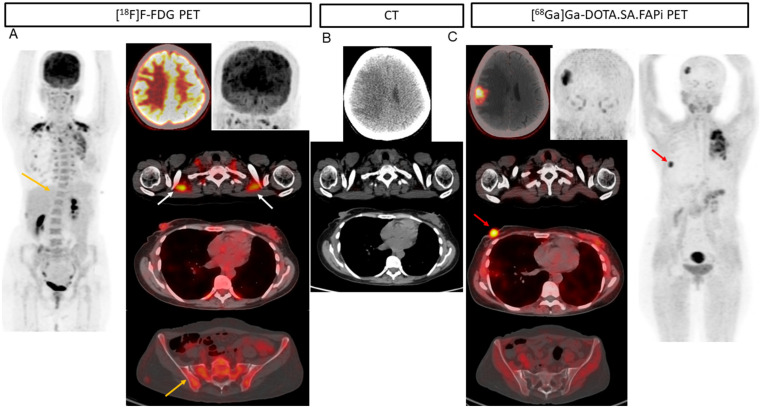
(A) 37-year-old patient with infiltrating ductal carcinoma underwent chemotherapy, Herceptin treatment, gamma knife for brain metastases, lapatinib, and capecitabine oral chemotherapy. A heterogeneous lesion measuring 1.7 × 1.4 cm was seen in the right parietal lobe associated with grade II perifocal vasogenic edema (B). (A) Due to the physiological uptake of FDG in the brain, lesions are not well demarcated (A). On [^68^Ga]Ga-DOTA.SA.FAPi PET scan, brain metastasis is appreciated in the MIP image itself (C). Brown fat uptake is seen on [^18^F]F-FDG ((A), white arrows), but no such findings were noted on [^68^Ga]Ga-DOTA.SA.FAPi PET scan (C). High-intensity uptake on [^68^Ga]Ga-DOTA.SA.FAPi in the right areola region corresponds to the mass in CT scan (B), but minimal radiotracer uptake on [^18^F]F-FDG PET scan (A). Diffuse non-specific bone marrow uptake was appreciated on [^18^F]F-FDG MIP (A) and transverse section ((A), yellow arrow) in the axial skeleton that was negative on CT (A,C) and [^68^Ga]Ga-DOTA.SA.FAPi (C) PET scans. [^68^Ga]Ga-DOTA.SA.FAPi clearly detected both primary and brain lesions on MIP itself (C).
